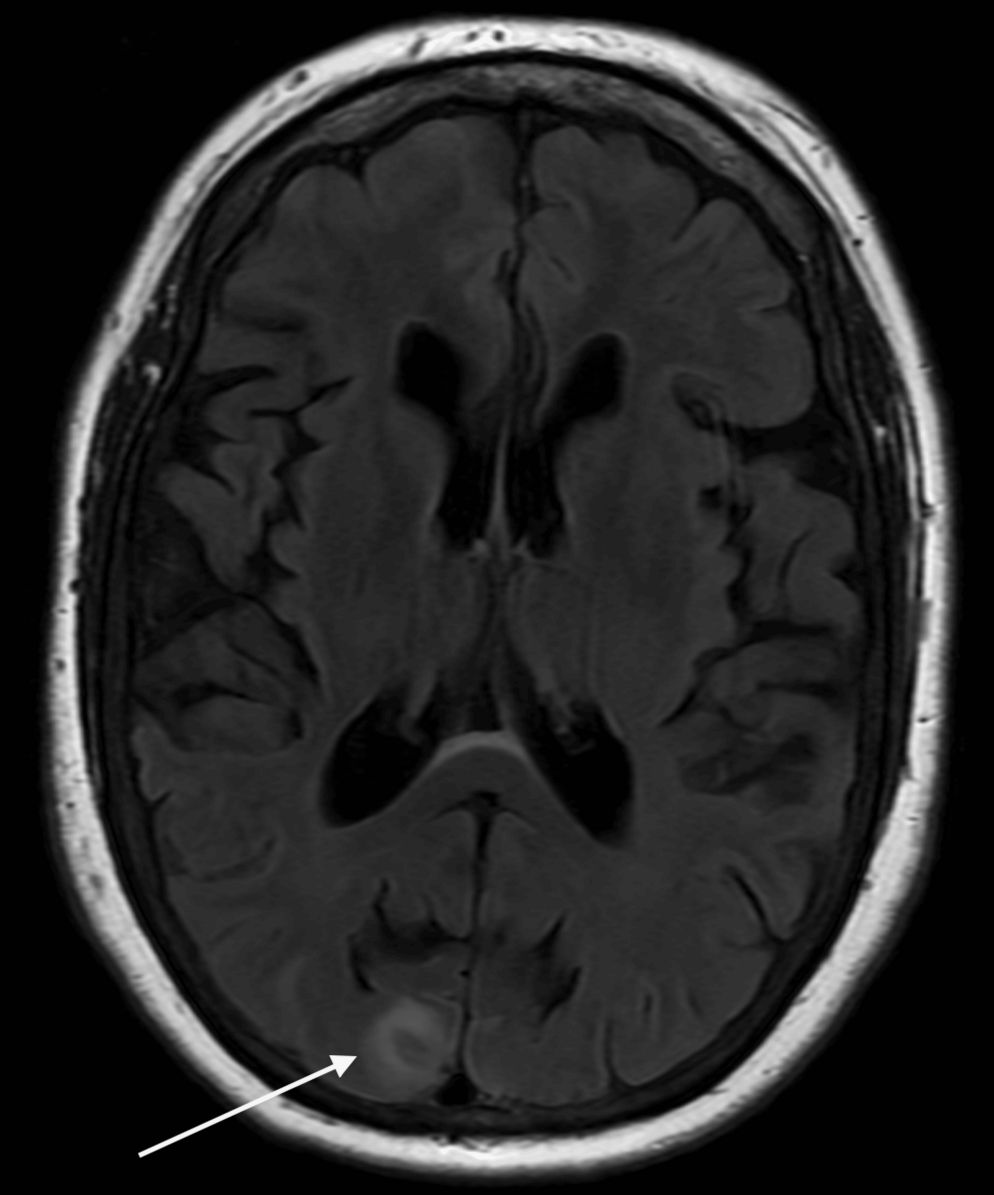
Axial T2 FLAIR MRI demonstrates a new cortically based hyperintense lesion in the right posterior occipital lobe (white arrow). This finding is consistent with parenchymal edema associated with ARIA-E, a known complication of anti-Aβ42 monoclonal antibody therapy. ARIA-E: amyloid-related imaging abnormalities with edema; FLAIR: fluid-attenuated inversion recovery
